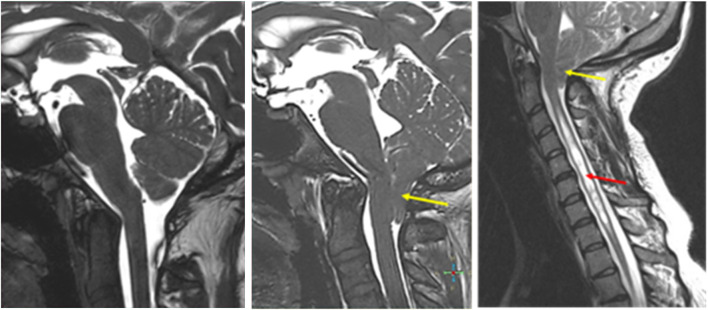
Midline sagittal MR images of a patent foramen magnum (left), tonsillar descent with obstruction of CSF flow (yellow arrow), and associated syringomyelia (red arrow).
